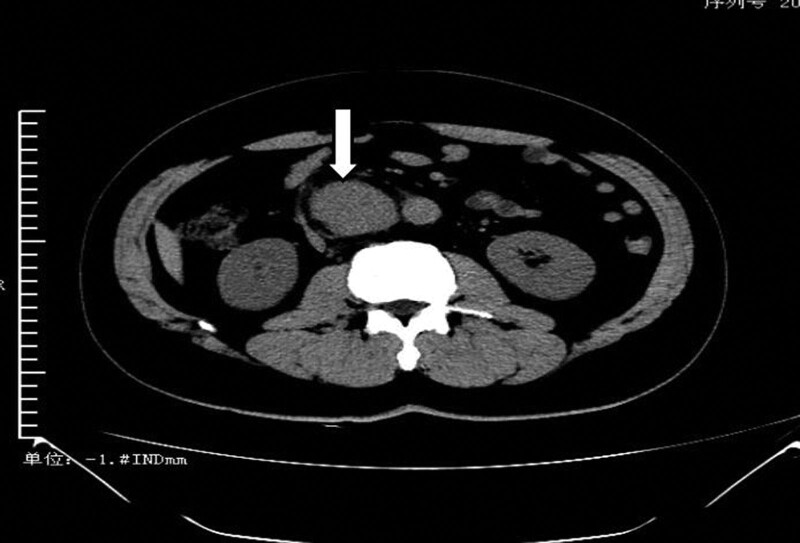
The CT scan revealed multiple nodular soft tissue shadows situated between the abdominal aorta and the inferior vena cava, with the largest measuring 5.0 * 4.0 cm. Additionally, there was compression and deformation of the inferior vena cava. CT = computed tomography.
